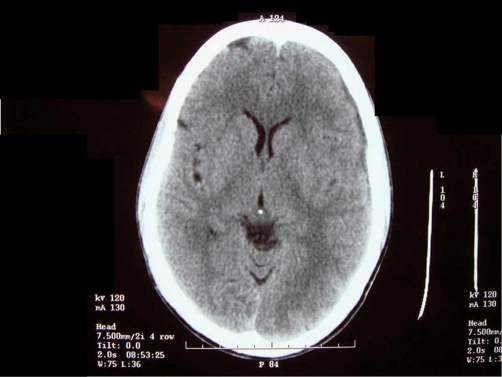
Photograph of the CT brain showing left minimal sub dural hygroma with no midline shift and no evidence of cerebral edema or cerebral atrophy.
